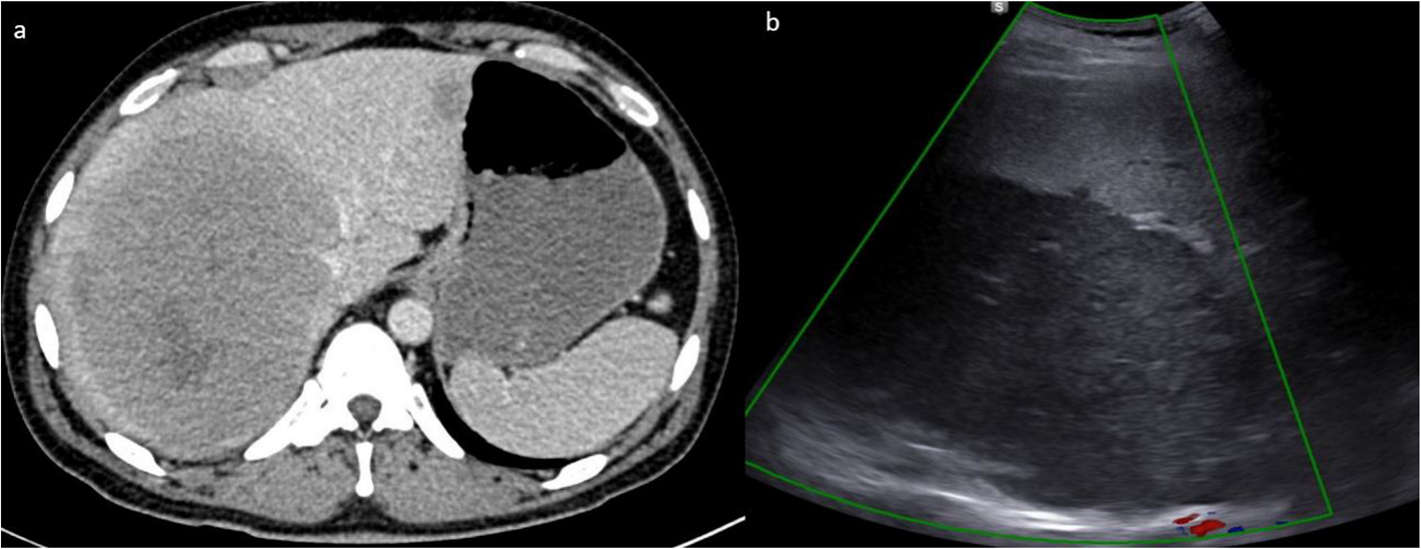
Axial CT images axial contrast-enhanced CT image and liver ultrasound of a patient with HIV-associated primary hepatic lymphoma. The contrast-enhanced CT shows a homogeneous soft tissue attenuation mass which does not show significant enhancement, and with fear preservation of the surrounding liver architecture. The mass displaces or encases the hepatic veins but does not invade vasculature or lead to tumor thrombus. On ultrasound, it appears as a fairly homogenous mass hypoechoic to the liver parenchyma. There is no increase vascularity within the mass or in its periphery
